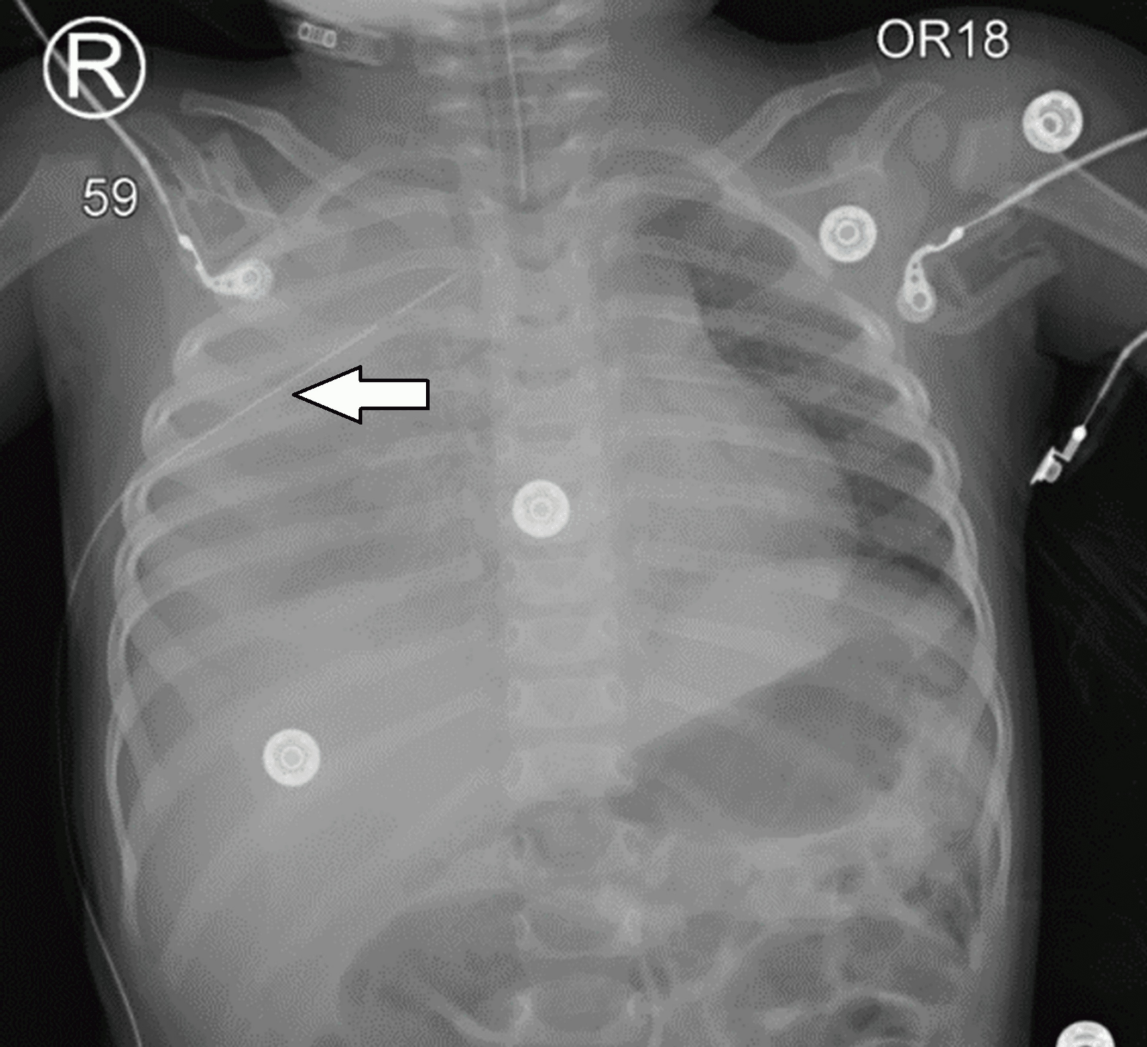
Anteroposterior chest X-ray showing right-sided hemothorax drainage with chest tube placement (white arrow).
